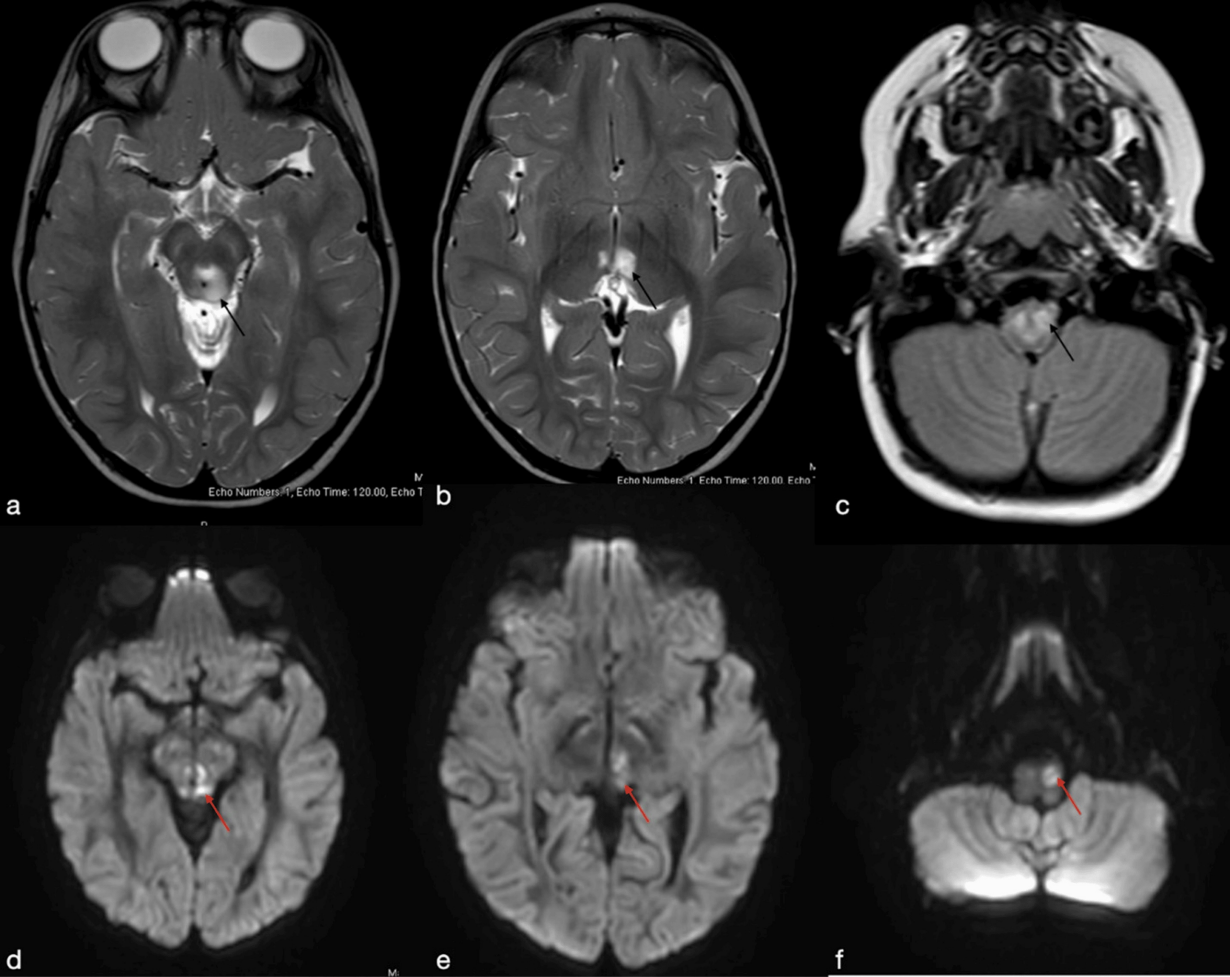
(a and b) T2-weighted axial images showing increased periaqueductal and medial thalami signal respectively (black arrows). (c) FLAIR axial imaging shows increased signal in the upper cervical spine (black arrow). (d-f) Diffusion-weighted imaging shows restricted diffusion in the periaqueductal, medial thalami, and inferior olivary nuclei respectively (red arrows) FLAIR: fluid-attenuated inversion recovery
